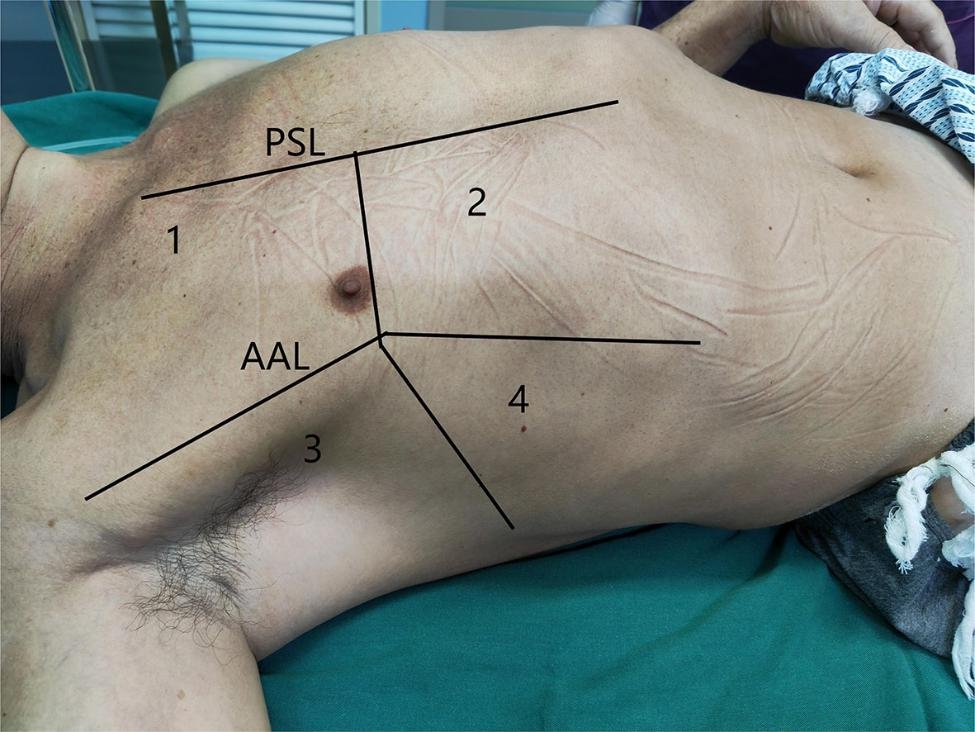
Eight-region lung subarea. Note: Parasternal line (PSL); anterior axillary line (AAL); ultrasound examination of the lungs divided into four quadrants: 1. Upper anterior lung area; 2: Anterior inferior lung area; 3. Superior lung area; 4. Inferior lung area
